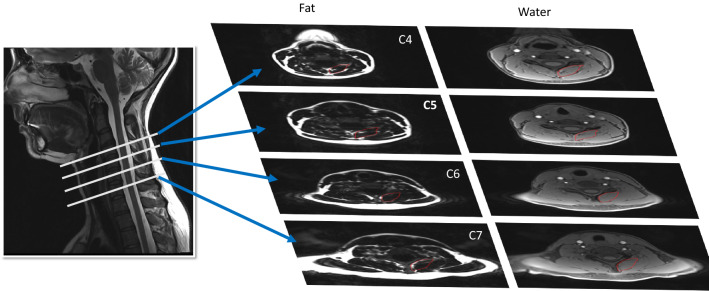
Fat/Water MRI of the deep cervical extensors from C4 through C7.
